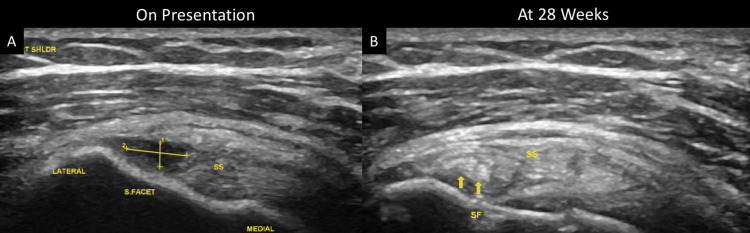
Ultrasound on presentation (A) and at 28 weeks (B). (A) Supraspinatus partial-thickness tear near tendon insertion site measuring 2.5 mm × 6.5 mm. (B) Resolved supraspinatus tear.
